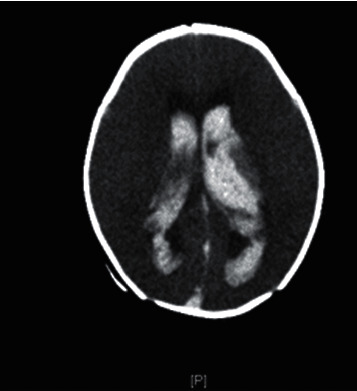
Computed tomography scan of the brain showing intraventricular hemorrhage involving the lateral third and fourth ventricles as well as the basal cistern with mild to moderate enlargement of the lateral ventricles.
